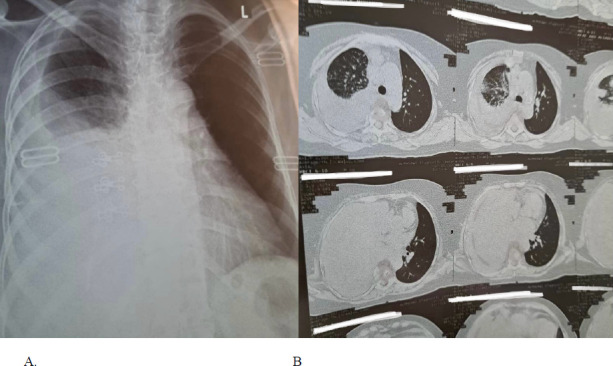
(A) Chest radiograph revealed a right-sided pleural effusion; (B) chest computed tomography was done to rule out any masses
